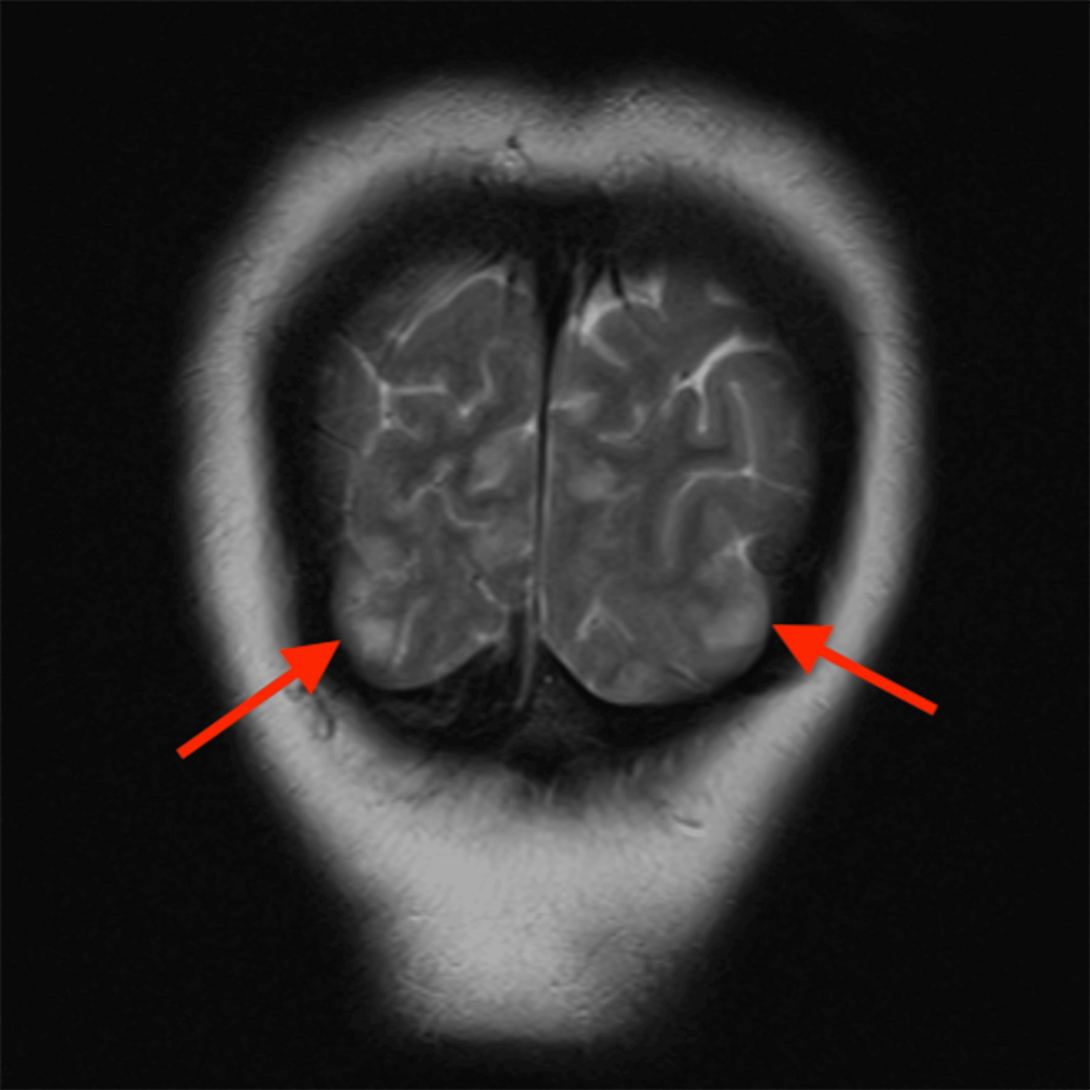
Coronal T2 MRI of Patient 1 (AS) demonstrating hyperintensities (arrows) in bilateral parietal regions The red arrows point to bilateral parietal regions with patchy, symmetrical cortical and subcortical T2 hyperintensities, consistent with PRES MRI: magnetic resonance imaging; PRES: posterior reversible encephalopathy syndrome
